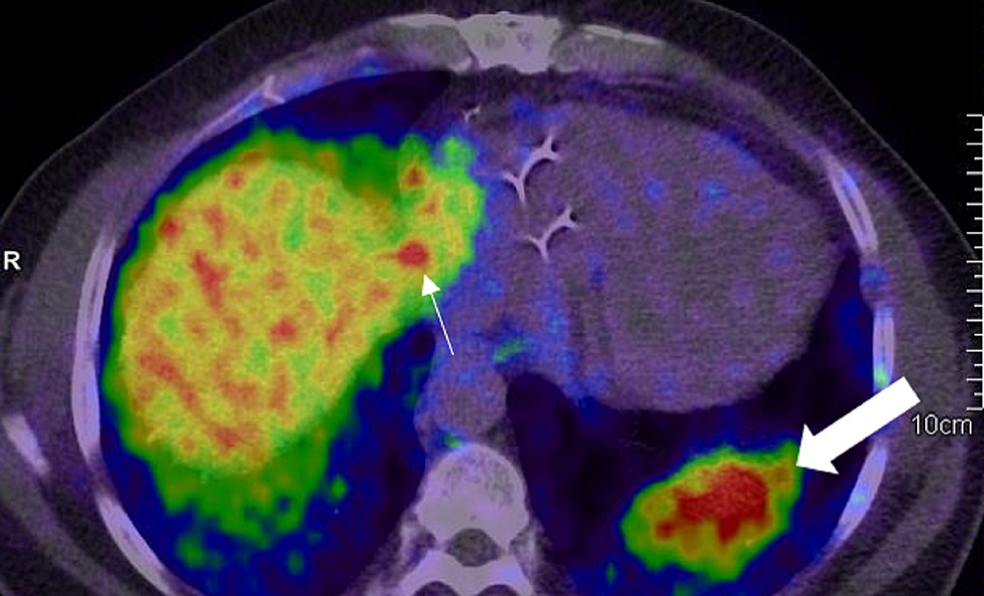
PSMA-RADS-3C Axial 68Ga PSMA PET/CT image of the upper abdomen shows a focal uptake in the left lobe of the liver (white thin arrow) that is indeterminate. There was no obvious correlate lesion on the low-dose CT. Further follow-up is required for clarification. Note the physiological uptake in the spleen (white thick arrow).
